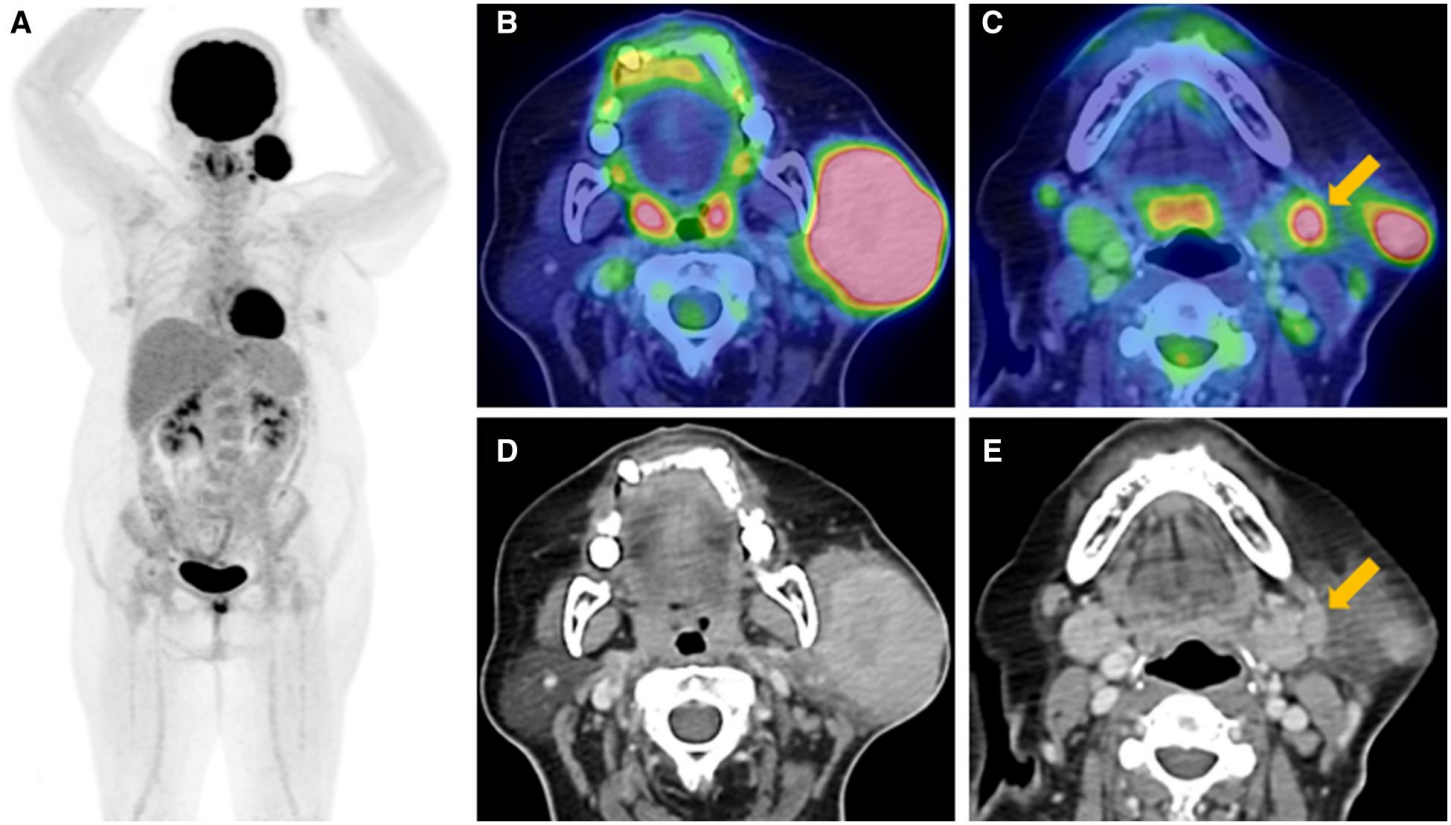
Fluorine-18 fluorodeoxyglucose PET CT ((A) maximum intensity projection; (B, C) axial hybrid; (D, E) axial CT) shows a hypermetabolic left parotid mass (B, D) and left cervical level Ib nodal metastases (C, E).
